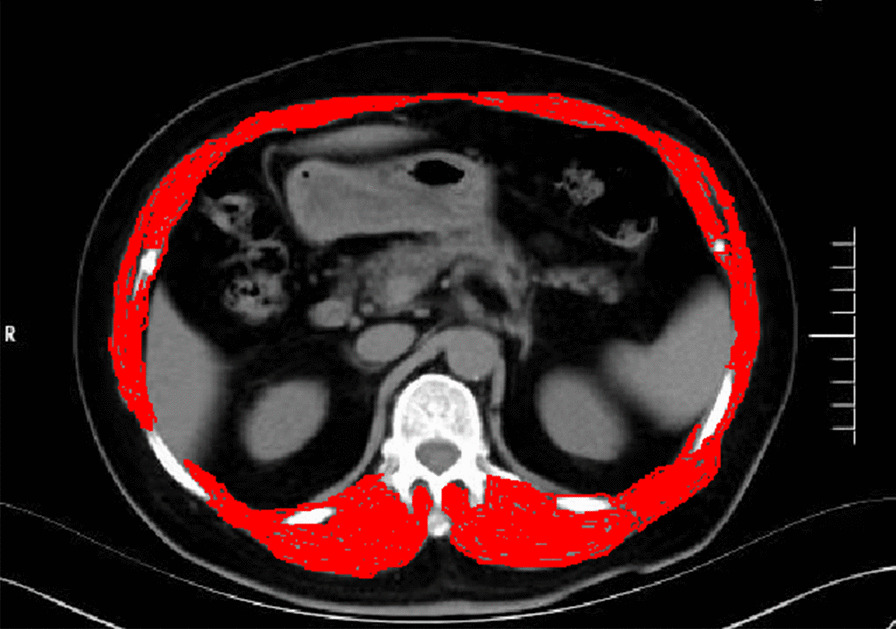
CT images used for the assessment of T12 SMI in OVCF patients. SMI was defined as the sum of the measured muscle area (at the level of the thoracic 12 vertebral body) divided by the square of the patient's height (cm^2^/m^2^). Muscle area was calculated by measuring muscle area including erector spinae, latissimus dorsi, internal abdominal oblique, external abdominal oblique, rectus abdominis, external intercostal muscles and intercostal muscles on CT images
